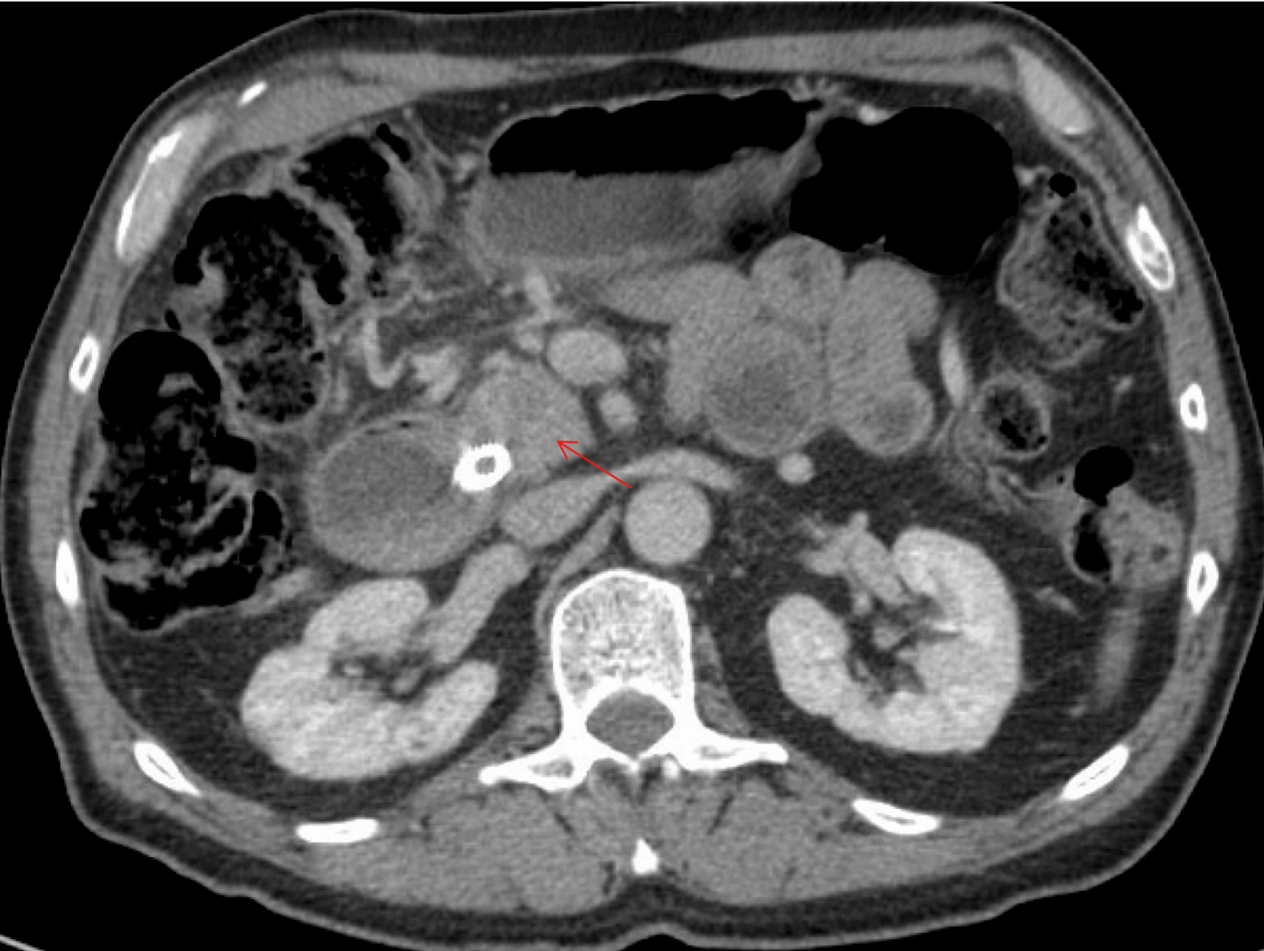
CT chest, abdomen, and pelvis, axial view in venous phase. The red arrow points at a hypoenhancing, rounded mass in the pancreatic head, abutting but not deforming the superior mesenteric vein.
